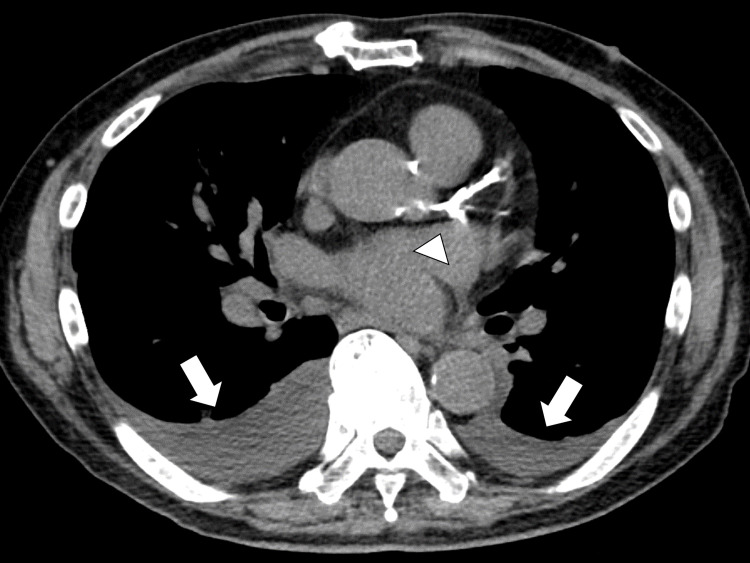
Chest CT showing bilateral pleural effusions (white arrow) and calcification of coronary arteries (white arrowhead) CT: computed tomography
